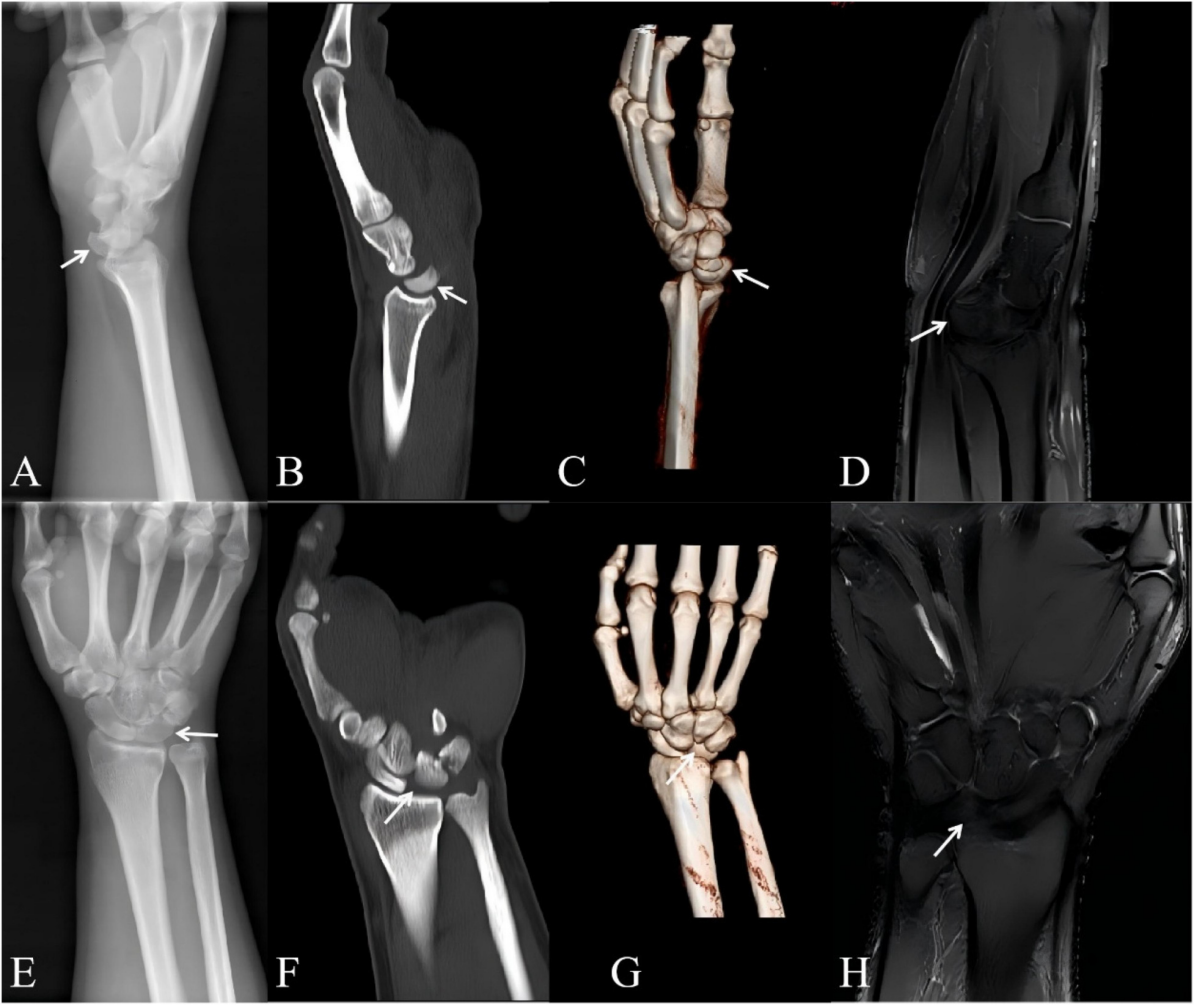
Preoperative imaging: x-ray: lateral radiograph (A) and posteroanterior radiograph (E); CT scan: sagittal view (B) and coronal view (F); 3D reconstruction: lateral view (C) and posteroanterior view (G); MRI: sagittal view (D) and coronal view (H) of the right wrist. White arrows indicate the volarly dislocated lunate.
